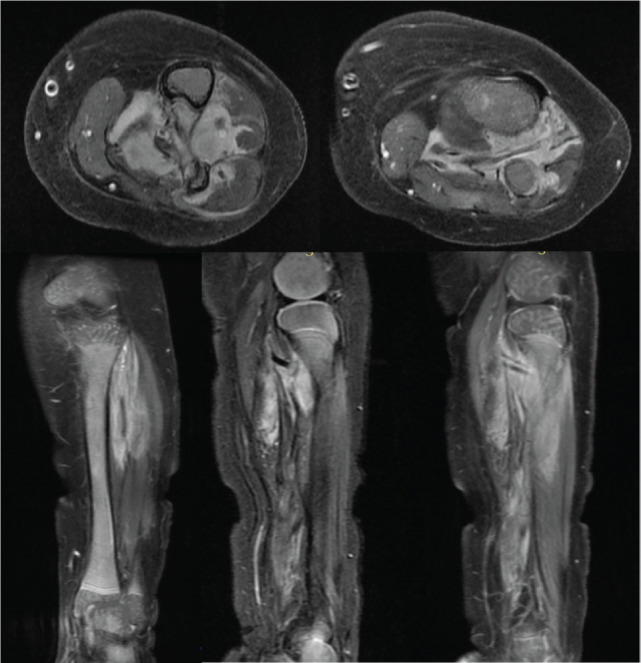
MRI scan images of the left leg showing local recurrence after wide local resection and 17 cycles of VAC-based chemotherapy.
